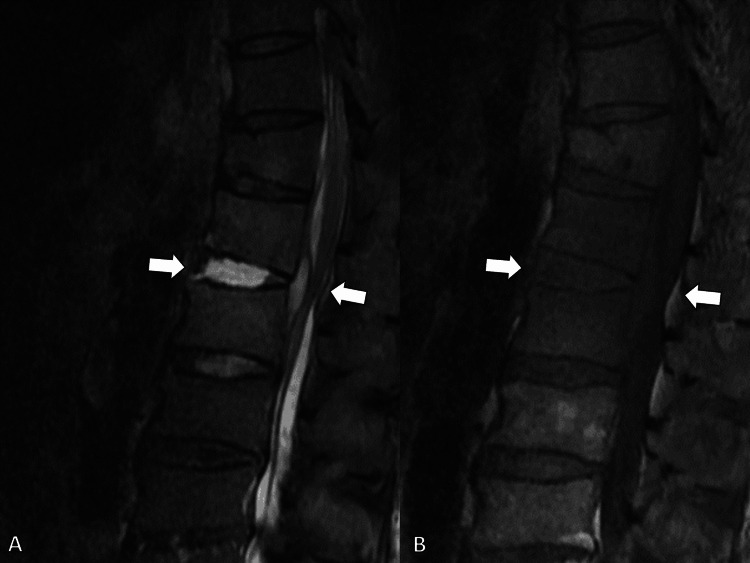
Magnetic resonance imaging of the lumbar spine showing dorsal epidural signal changes at L1/L2, consistent with an epidural abscess (white arrows). (A) Short τ inversion recovery image, and (B) T1-attenuated image.
